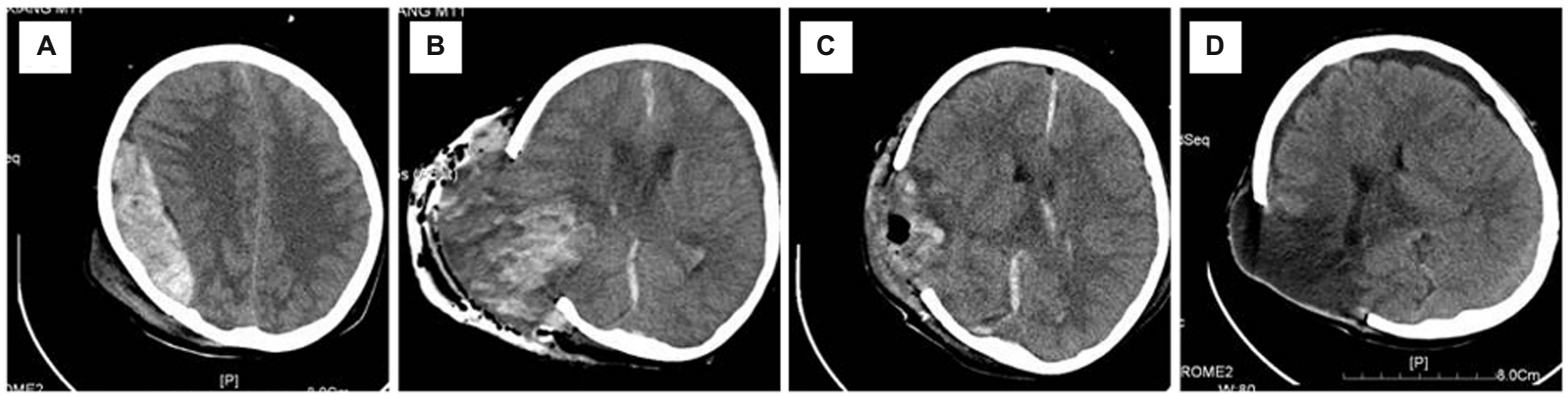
Representative radiographic images of Case 1. (A) An acute epidural hematoma in the right temporal–parietal area; (B) regional but not hemispherical bulging of the brain tissue occurred after hematoma evacuation, and intra-operative CT confirmed that there was no remote or contralateral hematoma except a “flame”-like intraparenchymal hemorrhage in the hematoma-compressed area; (C) incarcerated and necrotic cerebral parenchyma was resected, and the remaining brain tissue became atonic; (D) 1-month CT follow-up showed the presence of a focal encephalomalacia in the affected area and a contralateral subdural effusion, without any remote cerebral infarction. CT, computerized tomography.
